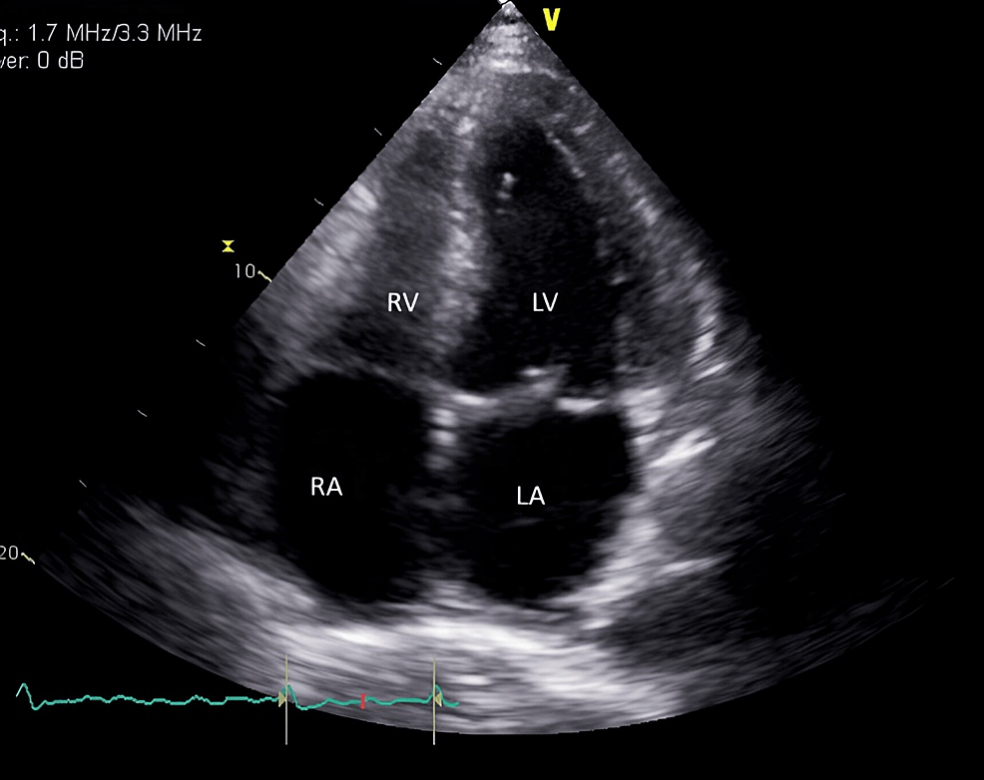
Echocardiogram of the patient showing improved regional wall motions RA, right atrium; LA, left atrium; LV, left ventricle; RV, right ventricle
